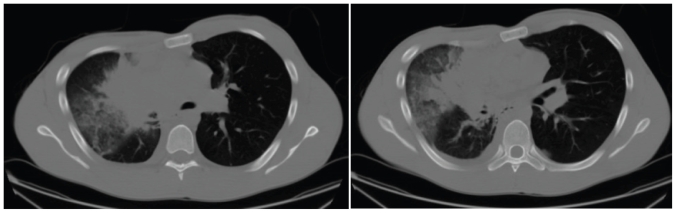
Computed tomography imaging of the thorax, with contrast, reveals a poorly defined 7×7×8-cm superior hilar mass.
